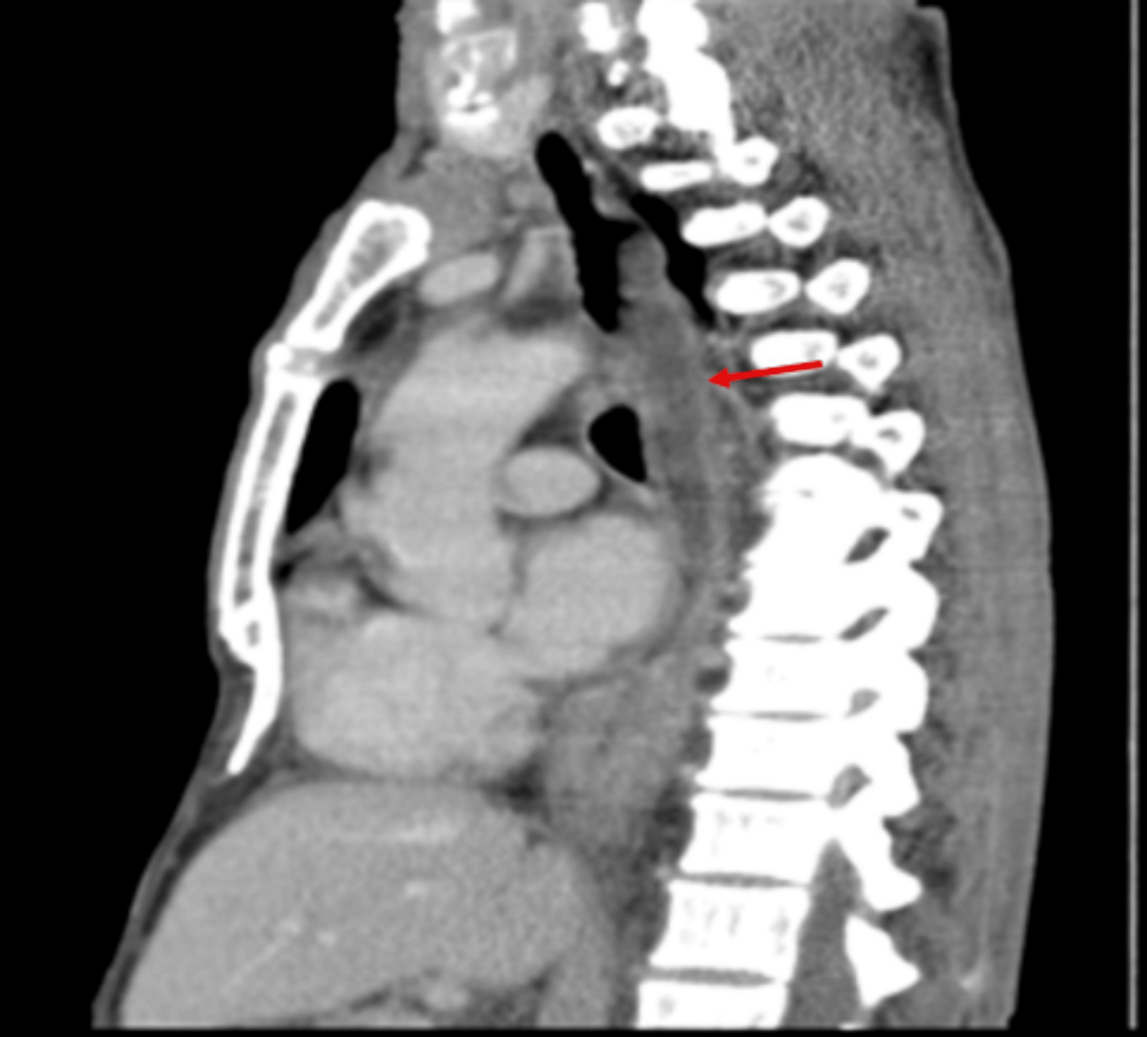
CT venous phase - sagittal section The red arrow shows the circumferential enhancing wall thickening of the distal esophagus.
